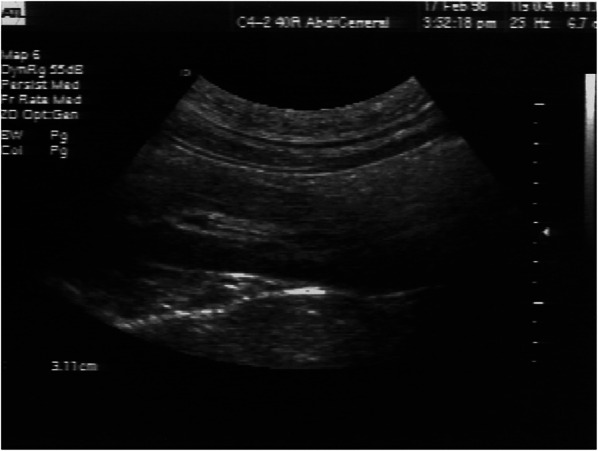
Sonographic view of the proximal ureter and renal pelvis with visualization of the echogenic guide wire and ureteral stent [32]
